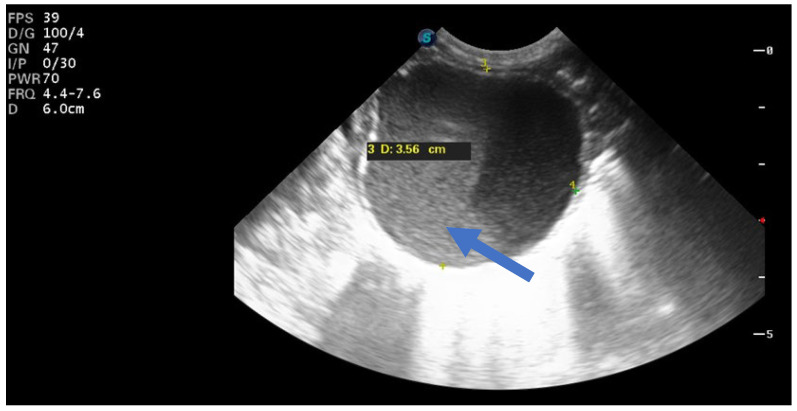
Ultrasound image of a simple cyst with an intracystic blood clot (arrow) at the age of 2 weeks postpartum, which regressed spontaneously at 2 months.
